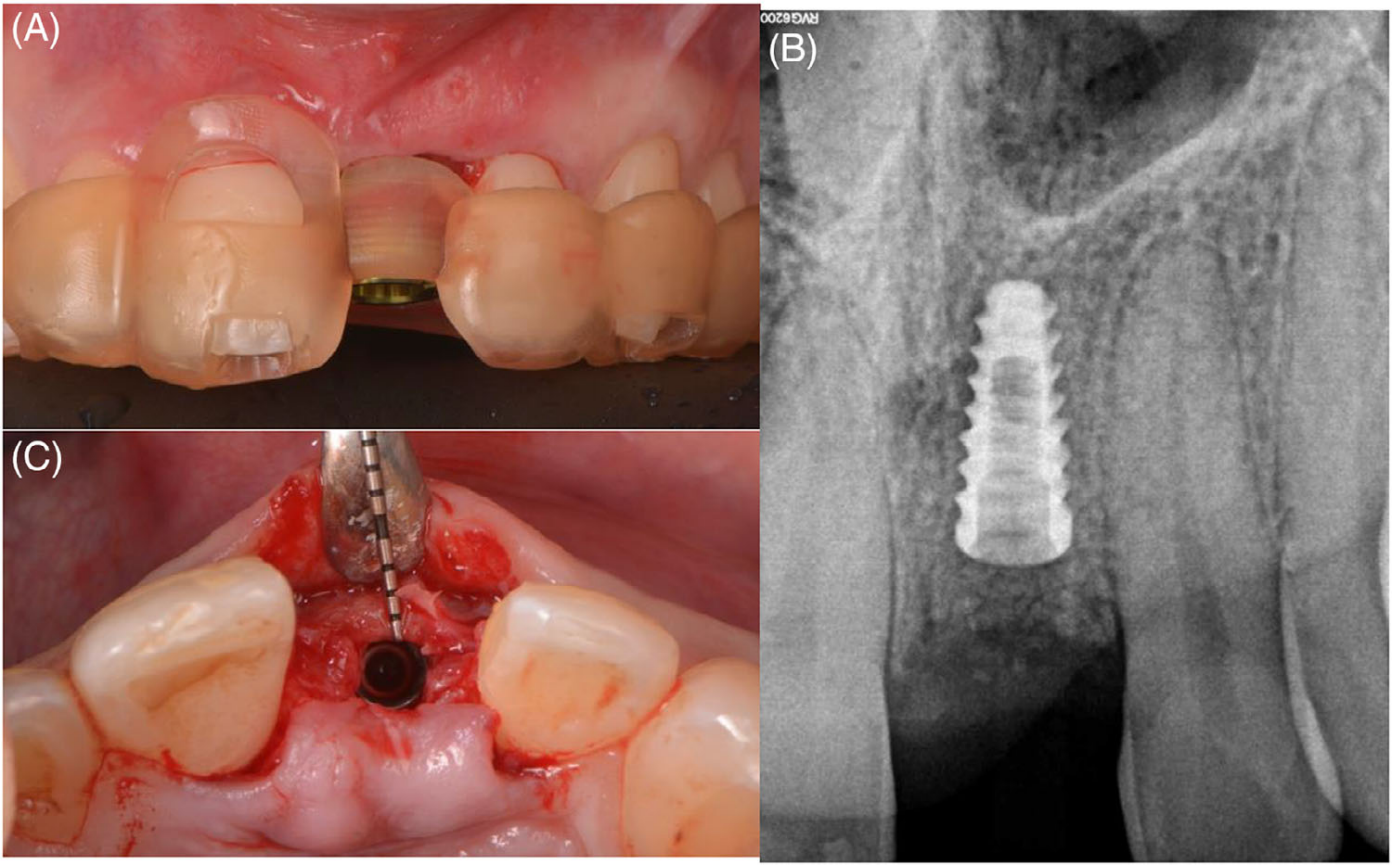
Re‐entry. Implant placement using a fully guided protocol (A). Critical buccal bone thickness present (C). Periapical radiograph post‐implant placement (B).
